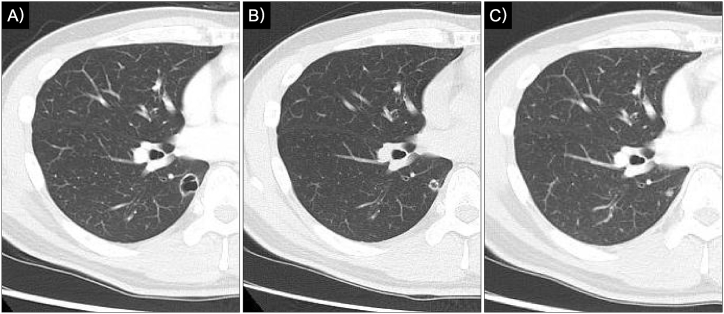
Chest CT findings A) At the time of examination: a cavitary lesion containing a 17-mm nodule. B) Cavity formation became apparent when the tumor measured approximately 7 mm. C) One year prior to evaluation at our department: a 4-mm nodule was present, with no evident cavity formation.
